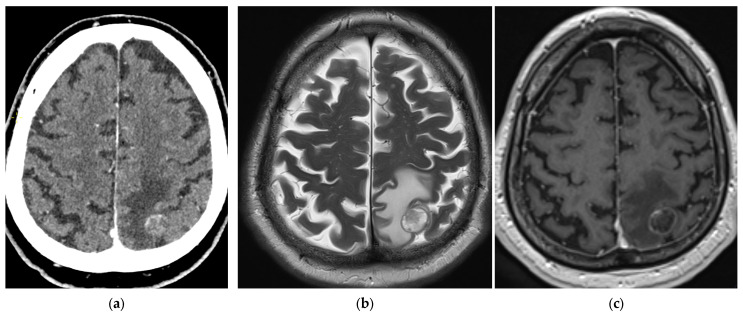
Brain metastasis: A single brain metastasis in the left parietal lobe in a patient with lung cancer indicates M1b disease. Axial post-contrast CT image (a), as well as T2-weighted (b) and post-contrast T1-weighted MRI images (c), showing a heterogeneous, rim-enhancing mass with a perifocal vasogenic edema.
